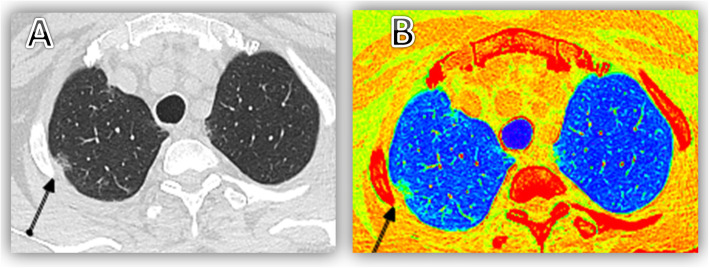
56 years old male patient presented with fever & chest symptoms including dry cough, yet CT chest was unremarkable (a) except for right upper lobe apical segment subpleural ill-defined faint small ground glass opacity, Which became more obvious in color coded images (b) (green in color)
